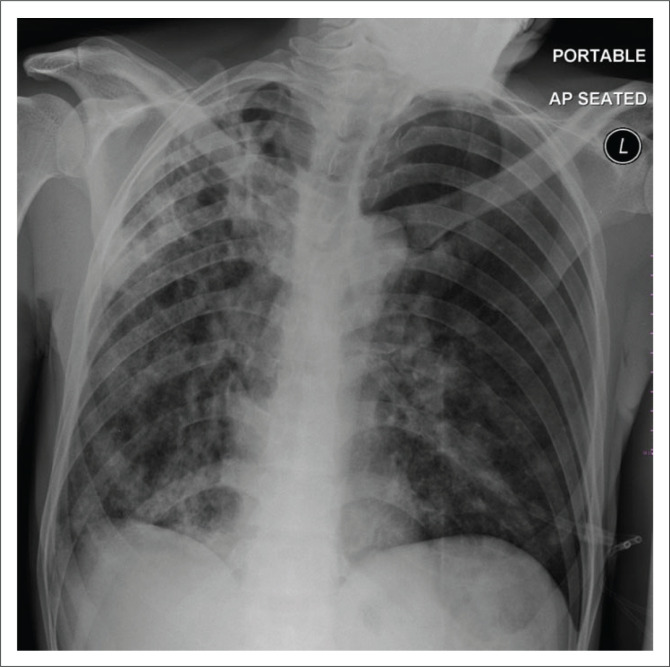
A 27-year-old male admitted to an isolation ward with COVID-19 pneumonia. Portable chest radiograph shows fibrosis and cavitation in the apical segment of the right upper lobe. Further bilateral right lung and left lingular air space opacities are noted. *Brixia* score: 15.
